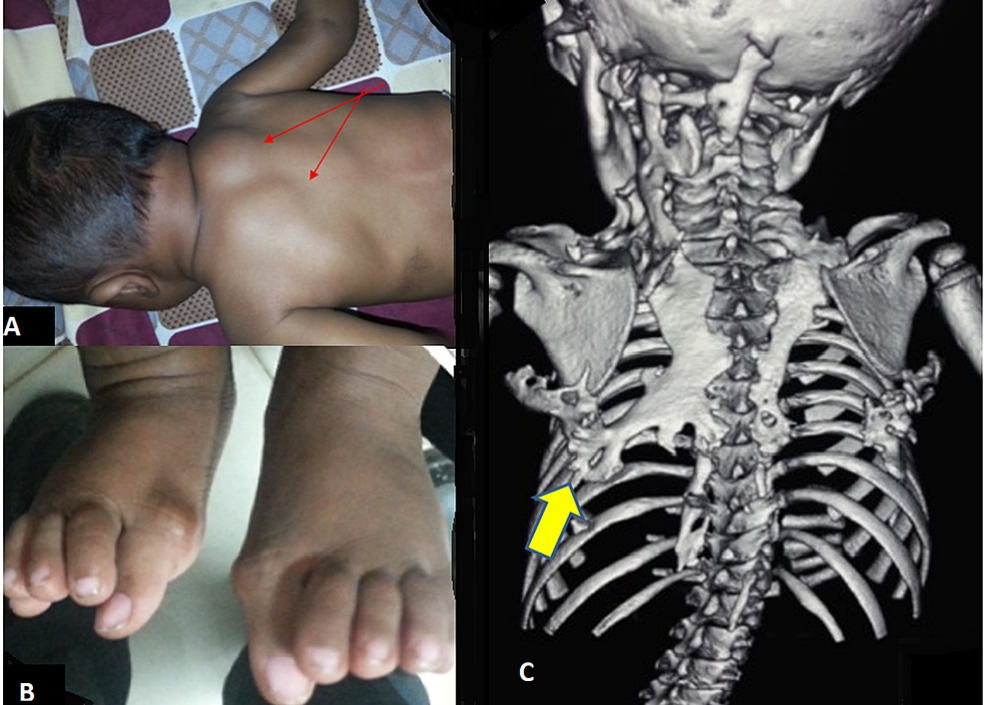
A seven months old female with pathognomonic clinical features of FOP: (A) heterotopic ossifications (red arrows) and (B) big toe deformity. (C) CT shows bony bridges (yellow arrow).
